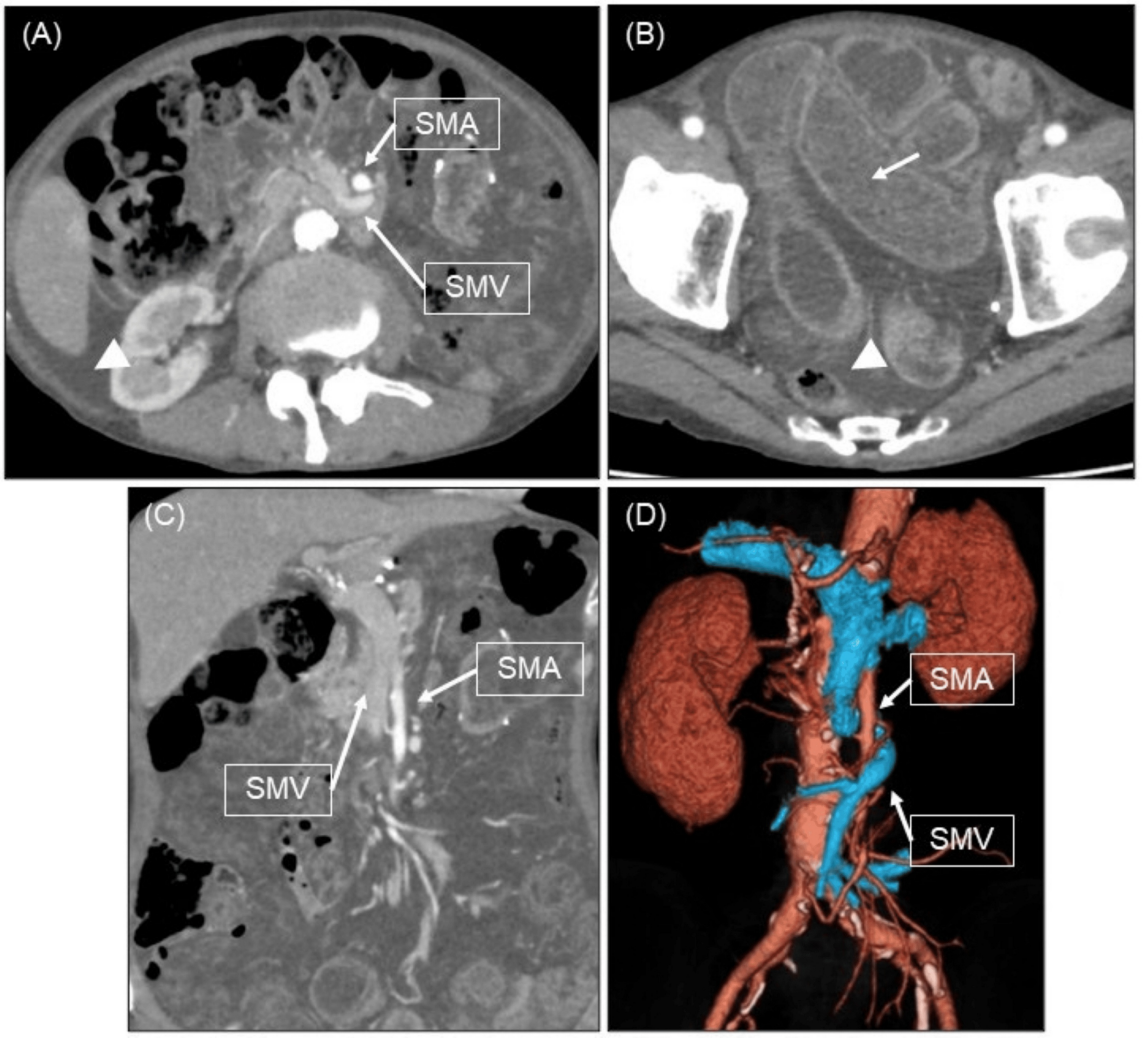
Contrast-enhanced CT and the CPR (A, B) Contrast-enhanced CT shows small bowel dilatation (white arrow) and ascites (white arrowhead). (C) Coronal section of contrast-enhanced CT. (D) The CPR definitively demonstrates a 360-degree torsion of the SMV around the main trunk of the SMA. CPR, curved planar reconstruction; SMV, superior mesenteric vein; SMA, superior mesenteric artery.
